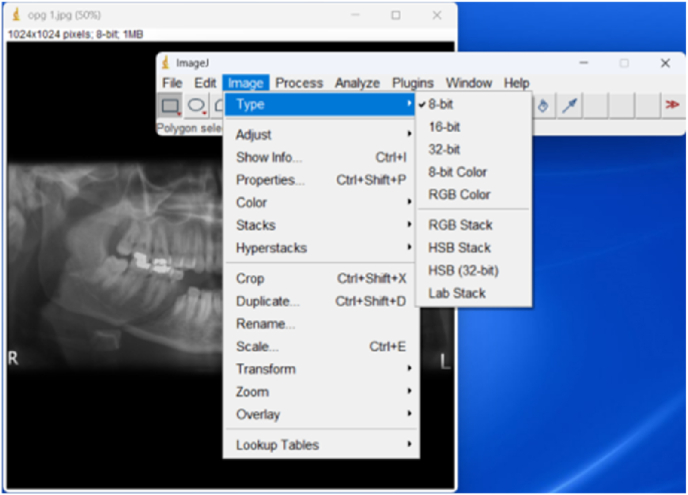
Adjusted Panoramic radiograph type in ImageJ.
